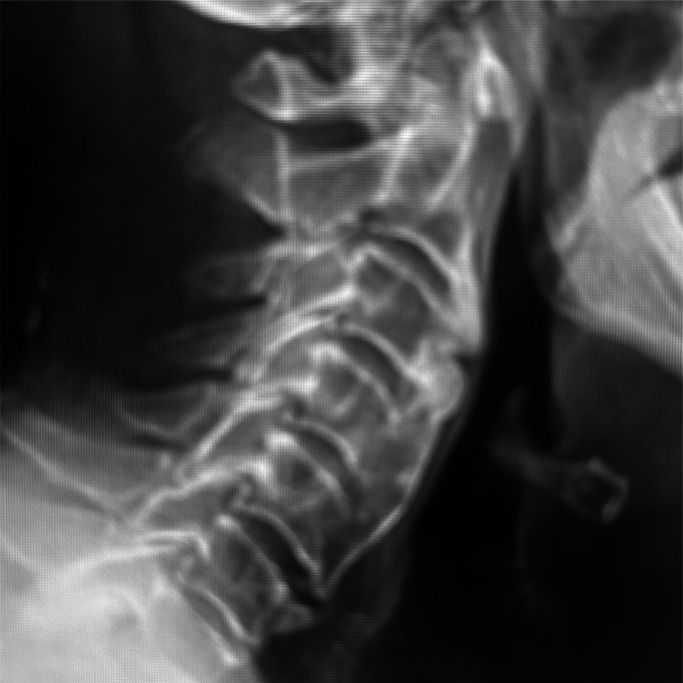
X-ray of the cervical spine of a patient with type 2 diabetes showing flowing anterior osteophytes over four contiguous vertebrae with preservation of intervertebral disc space without significant degenerative intervertebral disease. There is absence of facet and costovertebral joint ankyloses.
